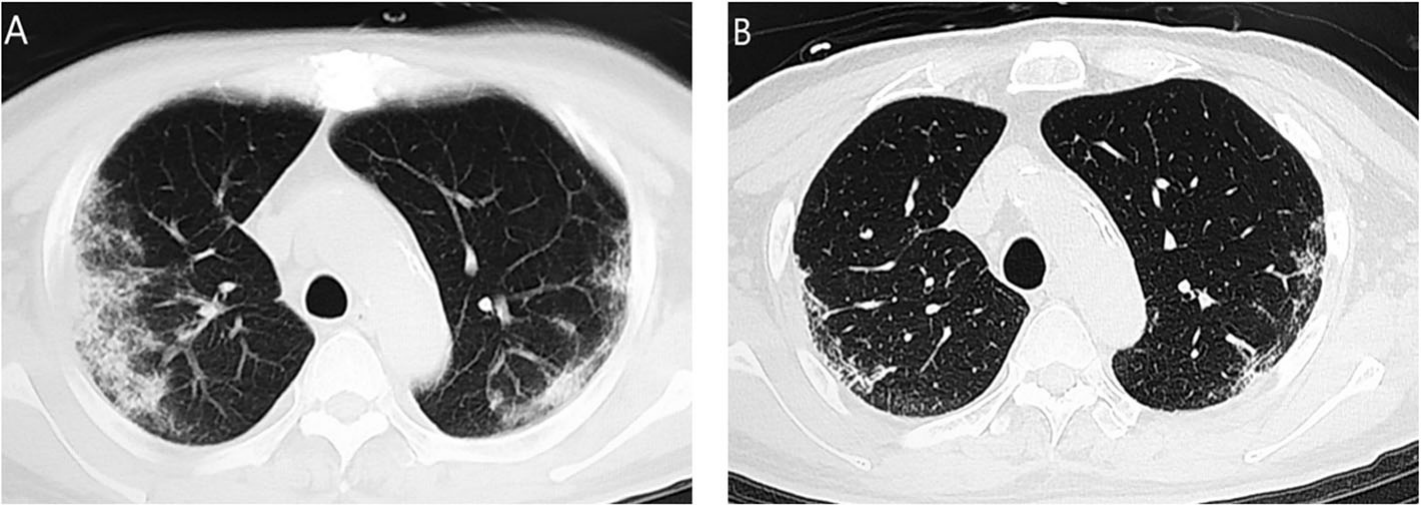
a CT scan obtained on the first day showed multifocal bilateral ground-glass opacities and consolidative pulmonary opacities. b CT scan obtained on day 12 showed that most of the lesions improved
